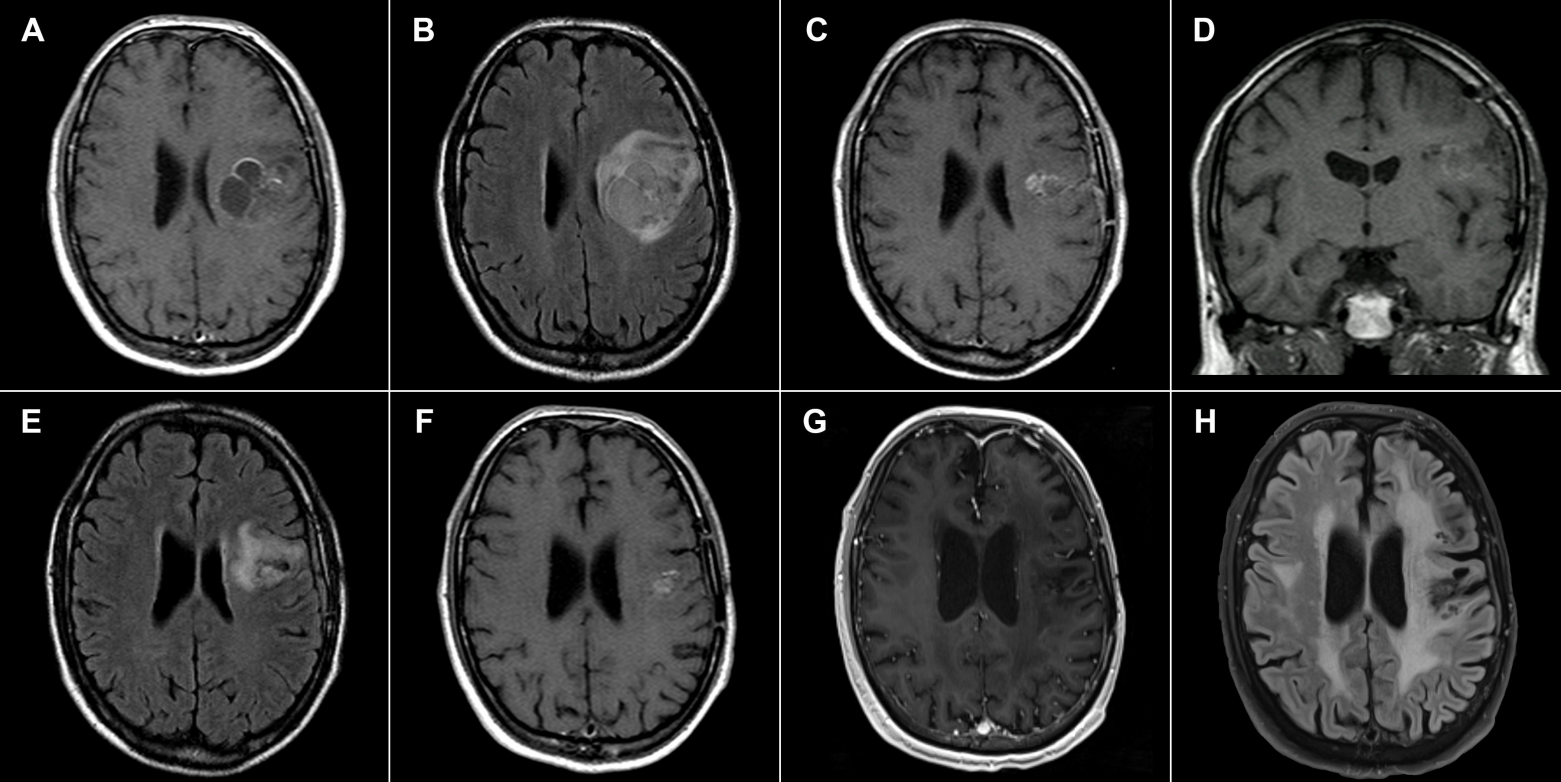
43-year-old male. (A) January 2004, 1 Tesla scanner: T1-weighted postcontrast spin echo preoperative image showing a GBM in the left central region. (B) Corresponding FLAIR image. (C) July 2004, 1 Tesla scanner: T1-weighted postcontrast spin echo postoperative image with a residual enhancing tumor after a partial resection (D) T1-weighted pre-contrast spin echo image from the same examination with minimal hyperintense signal at the border of the lesion (E) Corresponding FLAIR image. (F) August 2005 1 Tesla scanner: T1-weighted postcontrast spin echo image showing a decrease of the postcontrast enhancement suggesting a residual tumor regression. (G) May 2022, 3 Tesla scanner: T1-weighted postcontrast MP-RAGE sequence 217 months after surgery with no signs of residual tumor or its recurrence showing dystrophic and atrophic changes (H) Corresponding FLAIR image with dystrophic and post-radiation changes.
